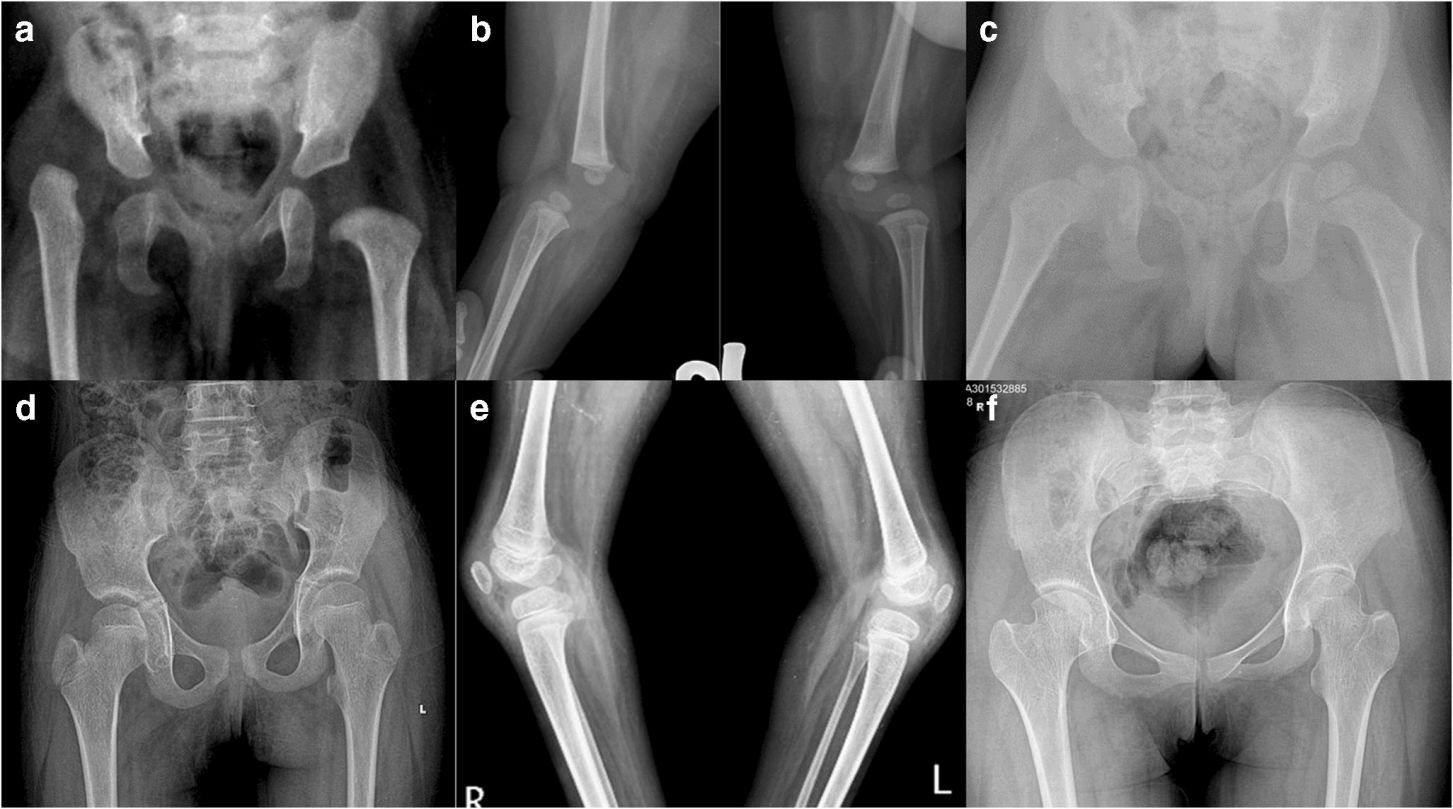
Serial radiographs of a child with arthrogryposis (a, b) who was operated at seven months for knee, and hip dislocation was reduced with open reduction at 2 years of age. Right acetabular dysplasia was persistent at six months from surgery (c). Excellent alignment of hip and knees at 14 years (d, e). She showed a good outcome of the hip at skeletal maturity with the remodeling of the right acetabulum (f).
